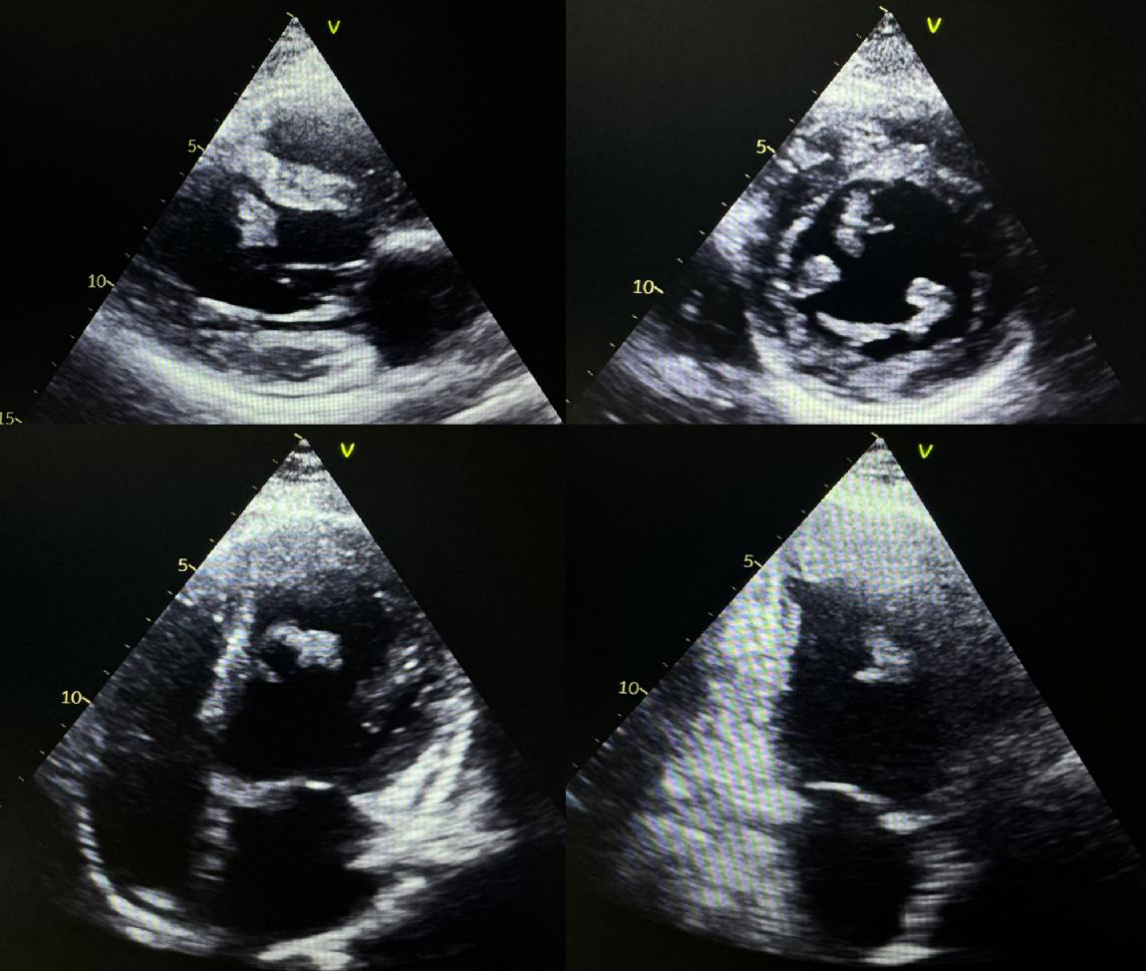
Transthoracic echocardiography images showing intra-LV thrombi.
